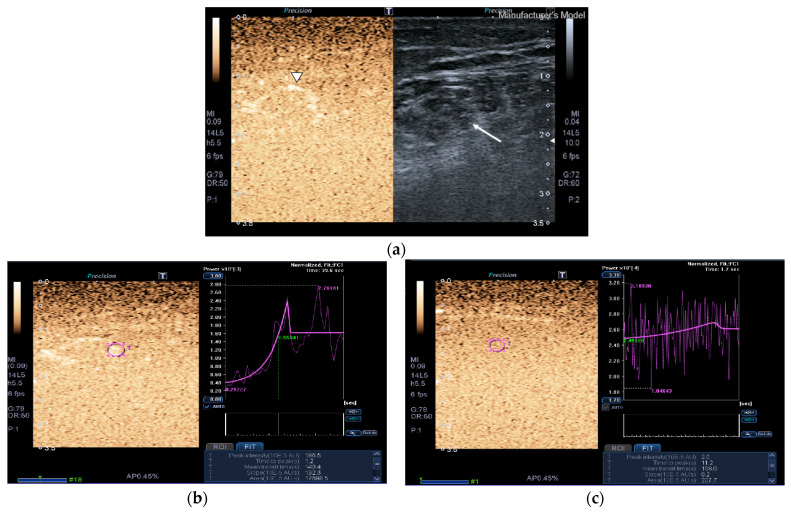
Transversal QRF muscle of a matched healthy control (85 years old). (a) Transversal ultrasonography scan shows the QRF muscle (arrow) and vascularization after contrast-enhanced ultrasound (CEUS) administration (arrowhead). CEUS image and time–intensity curve analysis are shown using the built-in software. (b) ROI (pink circle) in the area of strongest enhancement indicates maximum perfusion in the QRF. (c) ROI (pink circle) in the area of lowest enhancement indicates minimum perfusion in the QRF.
